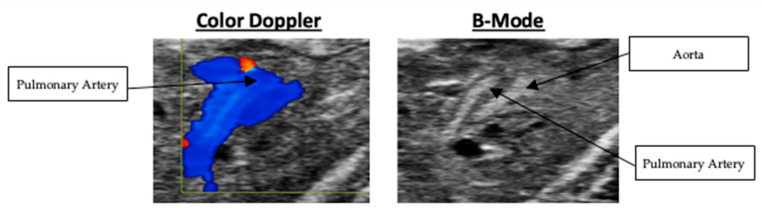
Color Doppler and B-Mode scans of pulmonary artery outflow velocity.
